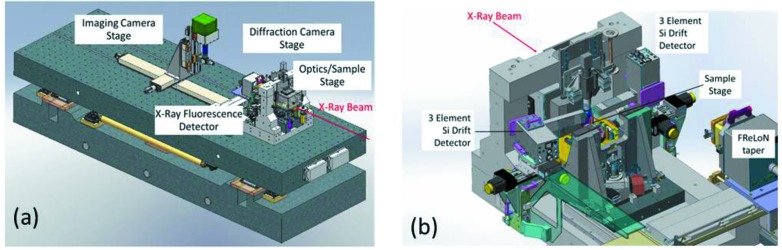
(*a*) Overview of the experimental table of ID16B end-station. (*b*) Amplified view of the nanoprobe. The X-ray beam is focused down to nanometer scale using KB optics located below the granite bridge structure.
